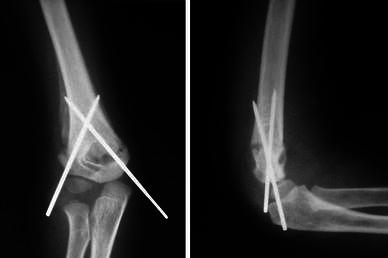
Follow-up radiograph at 3 weeks postsurgery showing fracture callus
